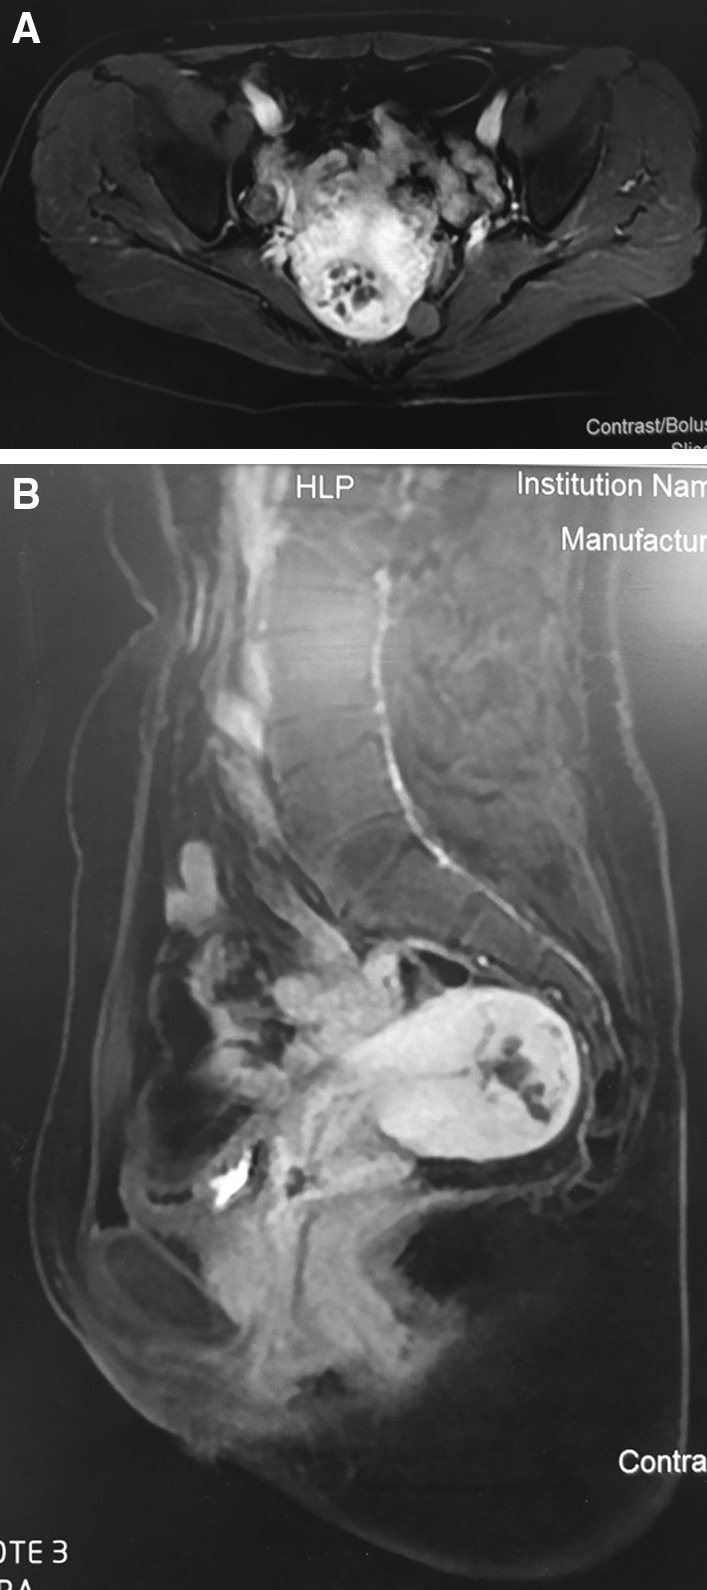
The pelvic MRI showed uterine lesion considered GTN, and abnormally increased blood vessels were detected in that lesion (A: MRI scan in axial view, B: MRI scan in sagittal view)
